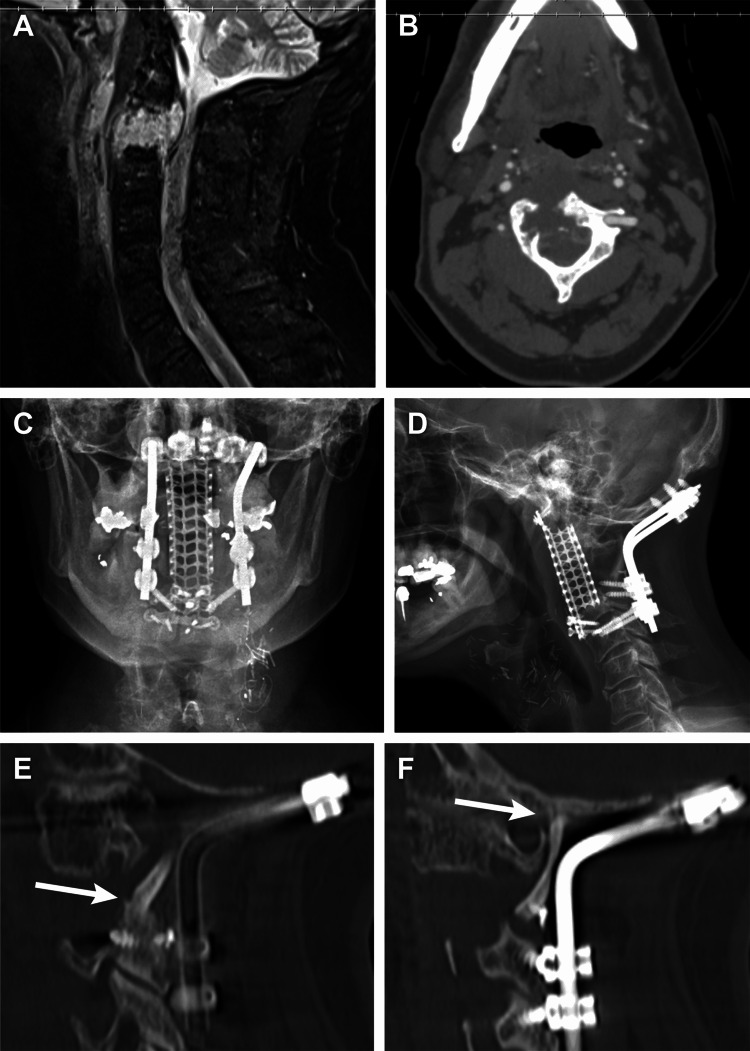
A man in his late 40s presented with a bony lesion at C2 that was found to have enlarged after three years of monitoring. (A) Preoperative sagittal MRI showed the lesion at C2 with epidural extension causing spinal cord compression. (B) Preoperative axial computed tomography (CT) showed a lytic bony lesion eccentric to the right side of C2. A transoral biopsy confirmed a pathologic diagnosis of chordoma, and the patient underwent a two-stage resection and reconstruction surgery. Posterior fixation was performed from the occiput to C4 with pedicle screws placed at the distal anchor point at C4 using the freehand technique with direct visualization. A second-stage anterior approach was then performed for en bloc tumor resection and anterior column reconstruction using a modified Harms cage and screws from the clivus to the C3 vertebral body. Three-month postoperative anterior-posterior (C) and lateral (D) radiographs showed intact hardware. (E-F) Six-month sagittal CT slices showed arthrodesis spanning the corpectomy (arrows). Used with permission from Barrow Neurological Institute, Phoenix, Arizona.
